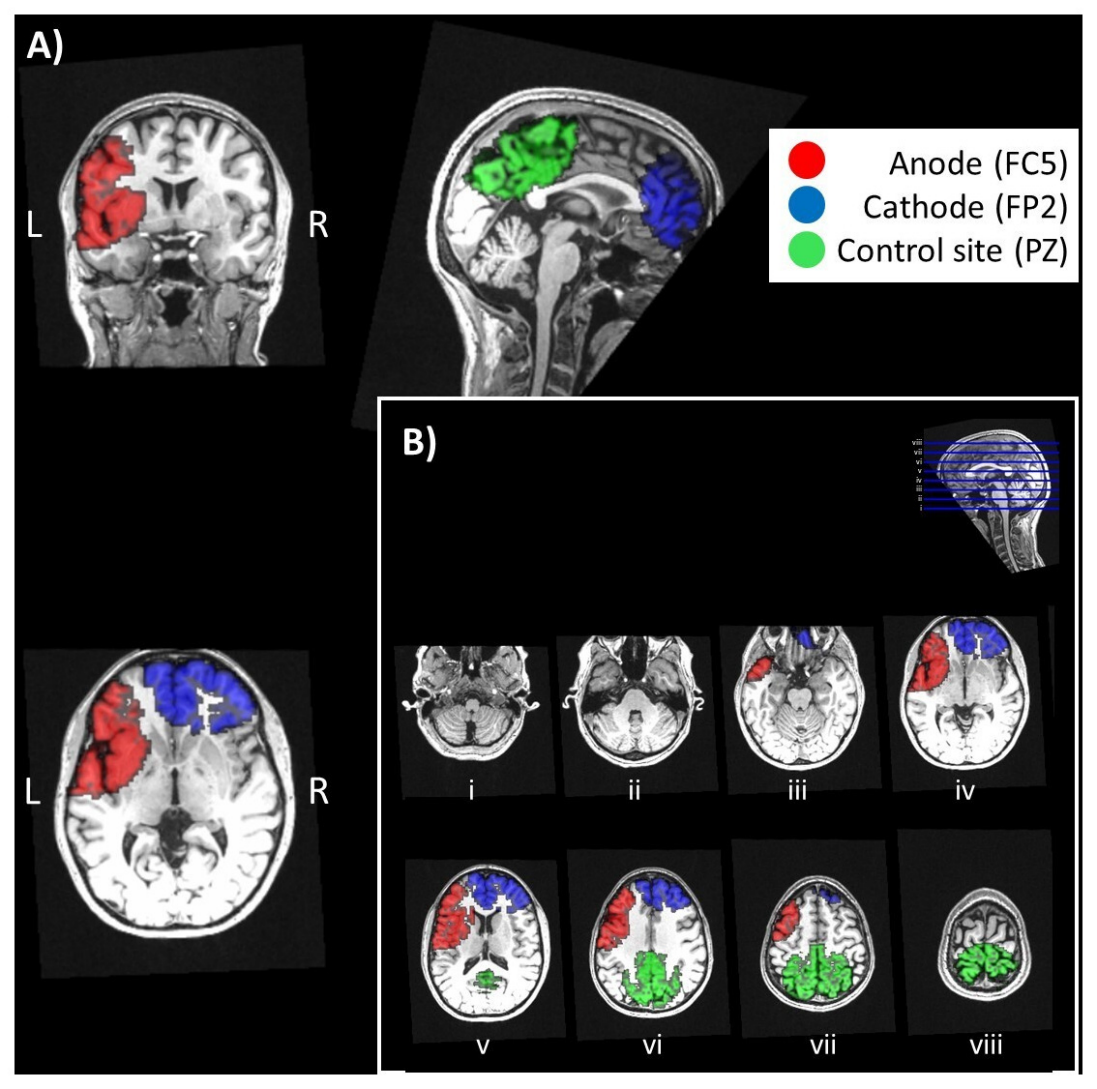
Regions-of-interest (ROIs). A) Locations of the three ROIs tested overlaid on the coronal, sagittal, and axial sections of a T1-weighted image. ROIs were defined beneath the anodal electrode (in red, corresponding to FC5 in a 10-20 system), cathodal electrode (in blue, corresponding to FP2) and at an independent site remote from the electrodes, used as a reference (in green, corresponding to PZ).
